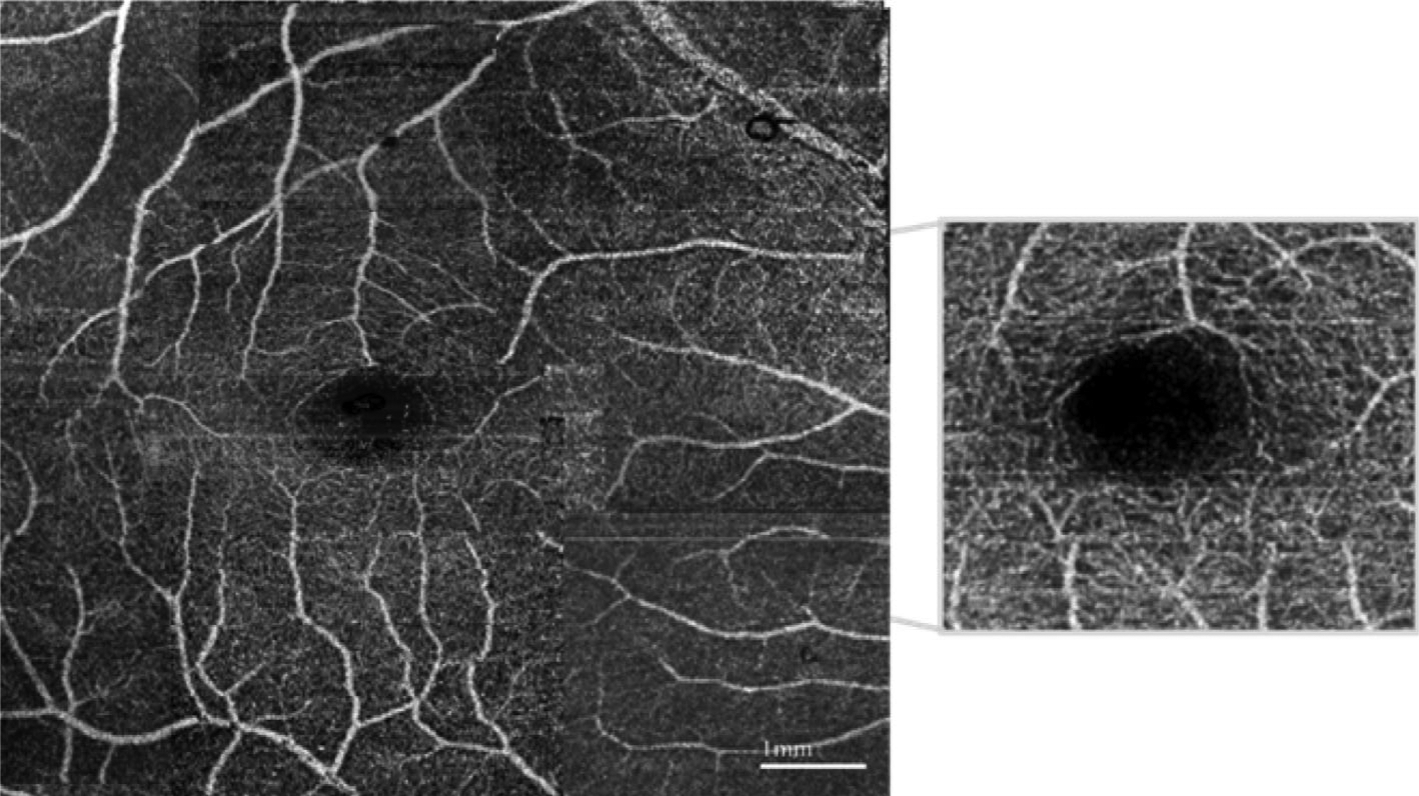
Retinal angiogram using intensity-based Doppler variance. Adapted from Reference [68]
